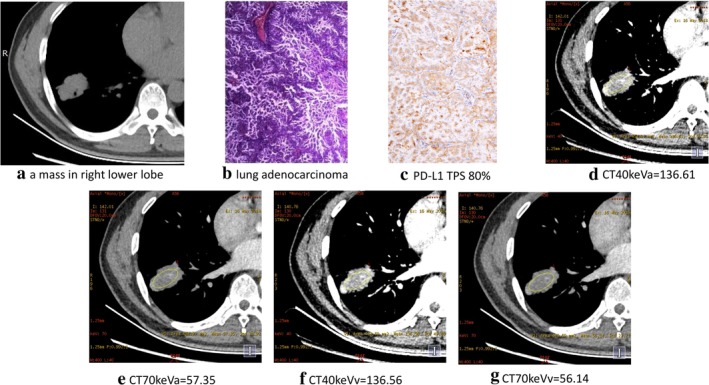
A 45‐year‐old male with right lower lung adenocarcinoma had 80% PD‐L1 TPS, on (a) conventional CT, (b) pathological section, (c) PD‐L1 expression, (d) CT40keVa, (e) CT70keVa, (f) CT40keVv and (g) CT70keVv.
